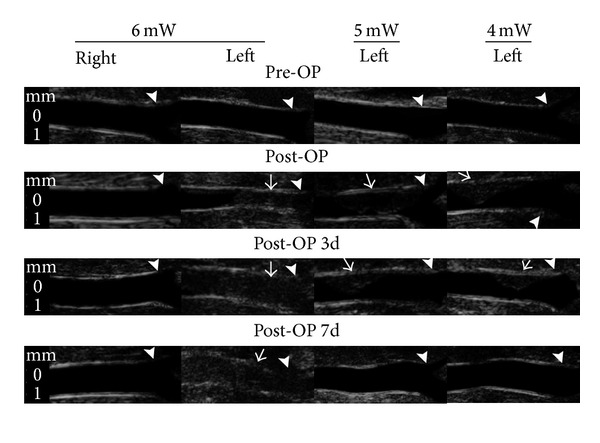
Ultrasound images of common carotid artery (CCA) after single LED irradiation with 6, 5, and 4 mW/cm^2^ for 4 h. The CCA is occluded immediately after 6 mW/cm^2^ LED irradiation. However, CCA is severely stenotic immediately after 4 and 5 mW/cm^2^ irradiation but is found to be recanalized at 7 days after irradiation. Pre-OP: before LED irradiation; post-OP: immediately after LED irradiation; post-OP 3 d: 3 days after LED irradiation; post-OP 7 d: 7 days after LED irradiation. The non-LED-irradiated right CCA is used as a control. White small arrowheads indicate the CCA bifurcation. White arrows demonstrate the formation of thrombus in the CCA.
